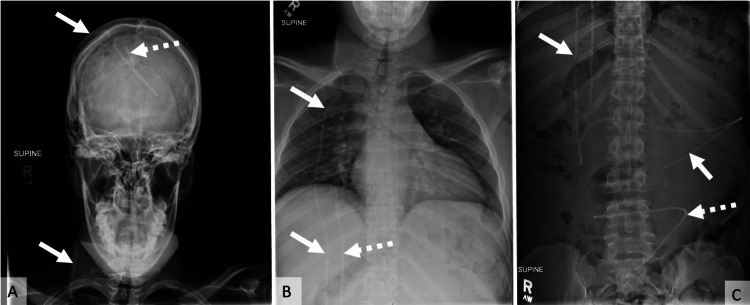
X-ray shunt series (A) Skull, (B) neck and chest, and (C) abdomen demonstrate an intact shunt catheter (solid white arrows) with a few abandoned catheters (dashed white arrows)
